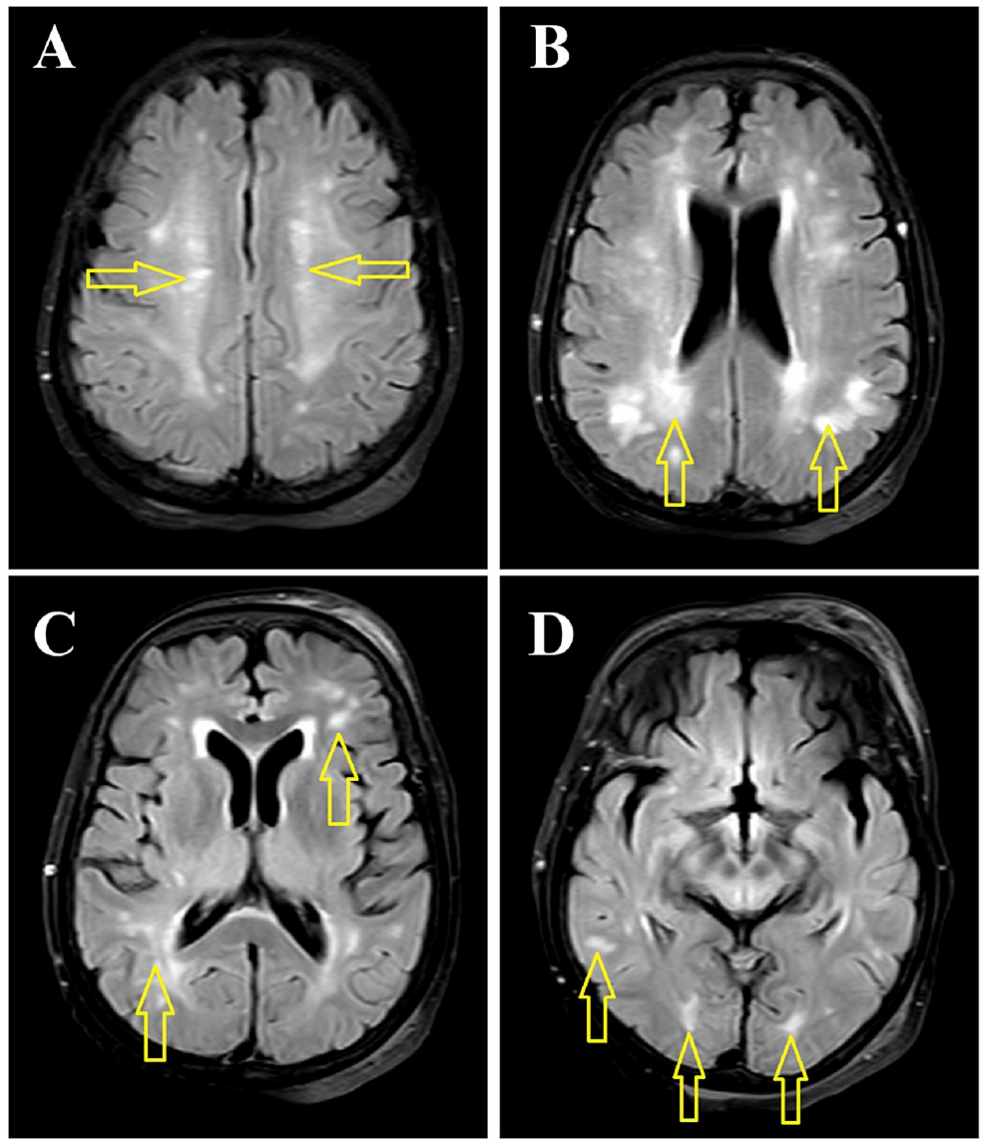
Axial FLAIR MR images (2A to 2D) of the brain at different levels show bilateral asymmetrical, discrete, and confluent hyperintense lesions (arrows) in the periventricular (1B, 1C) and subcortical white matter and centrum semiovale of both cerebral hemispheres (2A to 2D). FLAIR: fluid-attenuated inversion recovery; MR: magnetic resonance
